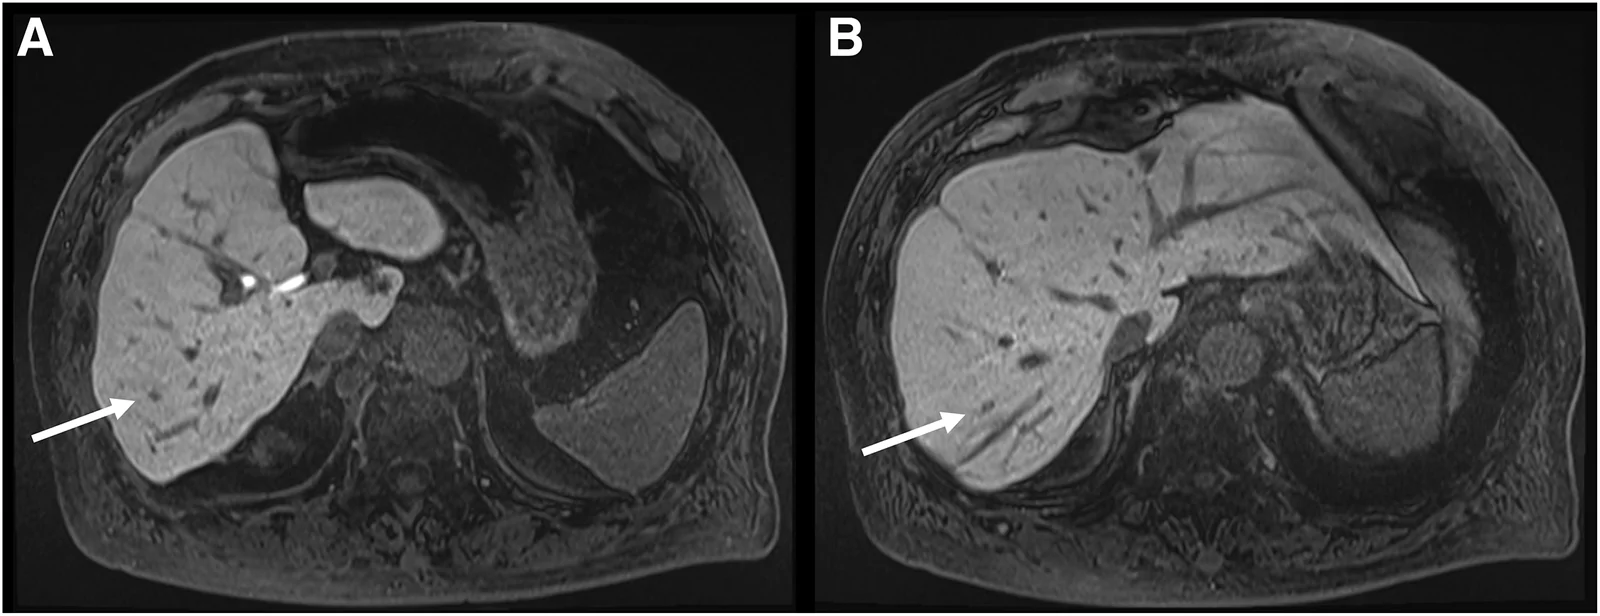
Liver Gd-EOB-DTPA–enhanced MRI in the hepatobiliary phase. (A) A space-occupying lesion with no Gd-EOB-DTPA uptake in S6 (arrow). (B) A space-occupying lesion with no Gd-EOB-DTPA uptake in S7 (arrow). Gd-EOB-DTPA, gadolinium ethoxybenzyl diethylenetriamine pentaacetic acid; S, segment
